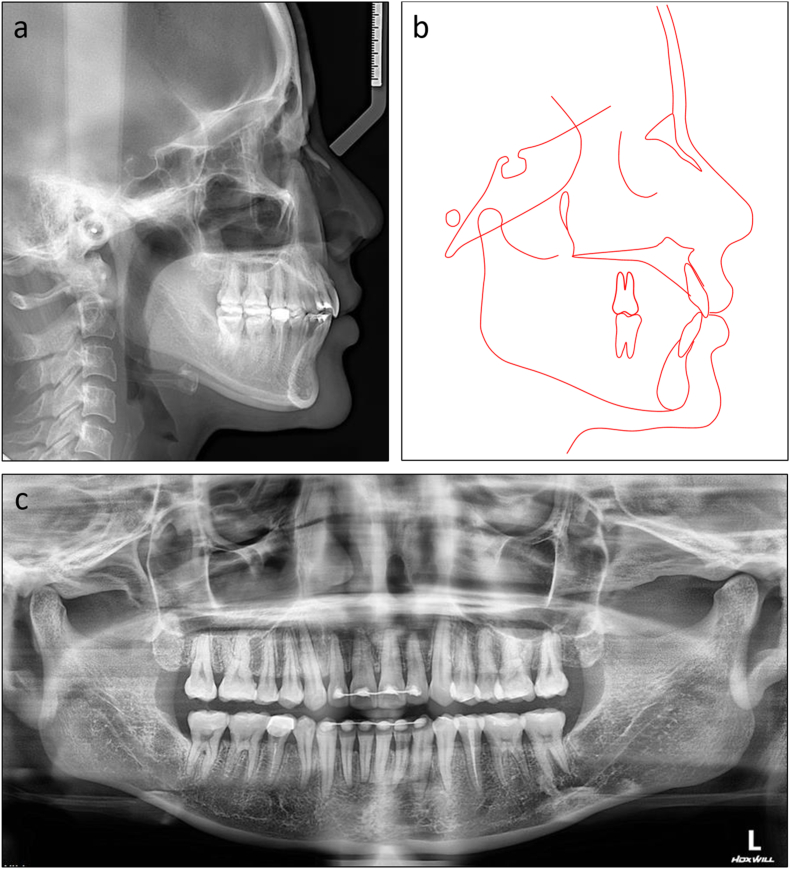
Post-treatment radiographs and cephalometric tracing of Case 1. (a) Cephalometric radiograph. (b) Cephalometric tracing. (c) Panoramic radiograph.
